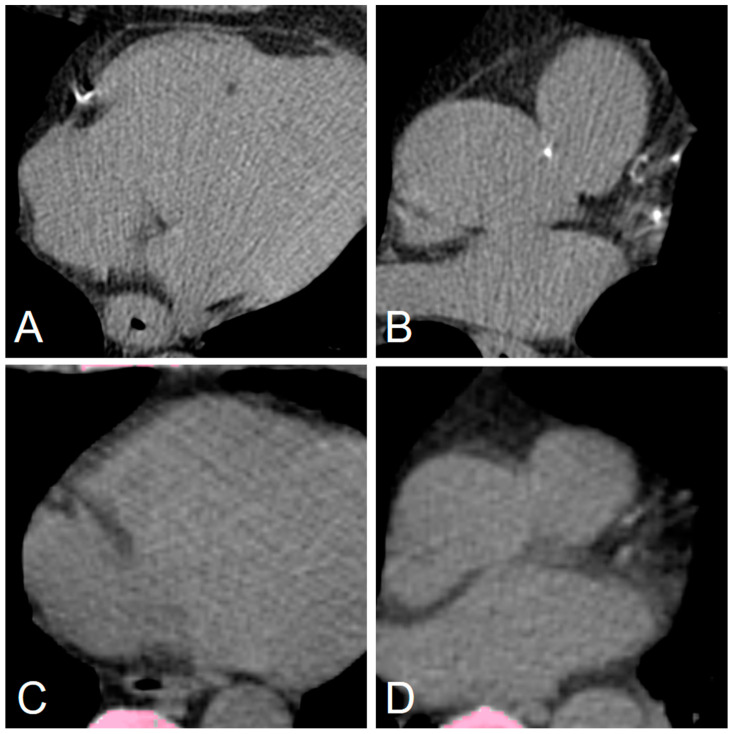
Representative false negative markings from the AI-CACS tool on ungated CT from PET/CT in a 73-year-old man undergoing PET/CT. Dedicated gated coronary calcium scan showed small coronary calcifications in the right coronary artery (A) and left anterior descending as well as left circumflex artery (B). However, the calcifications are not depicted on ungated low dose PET/CT (C,D) performed for staging of lung cancer.
